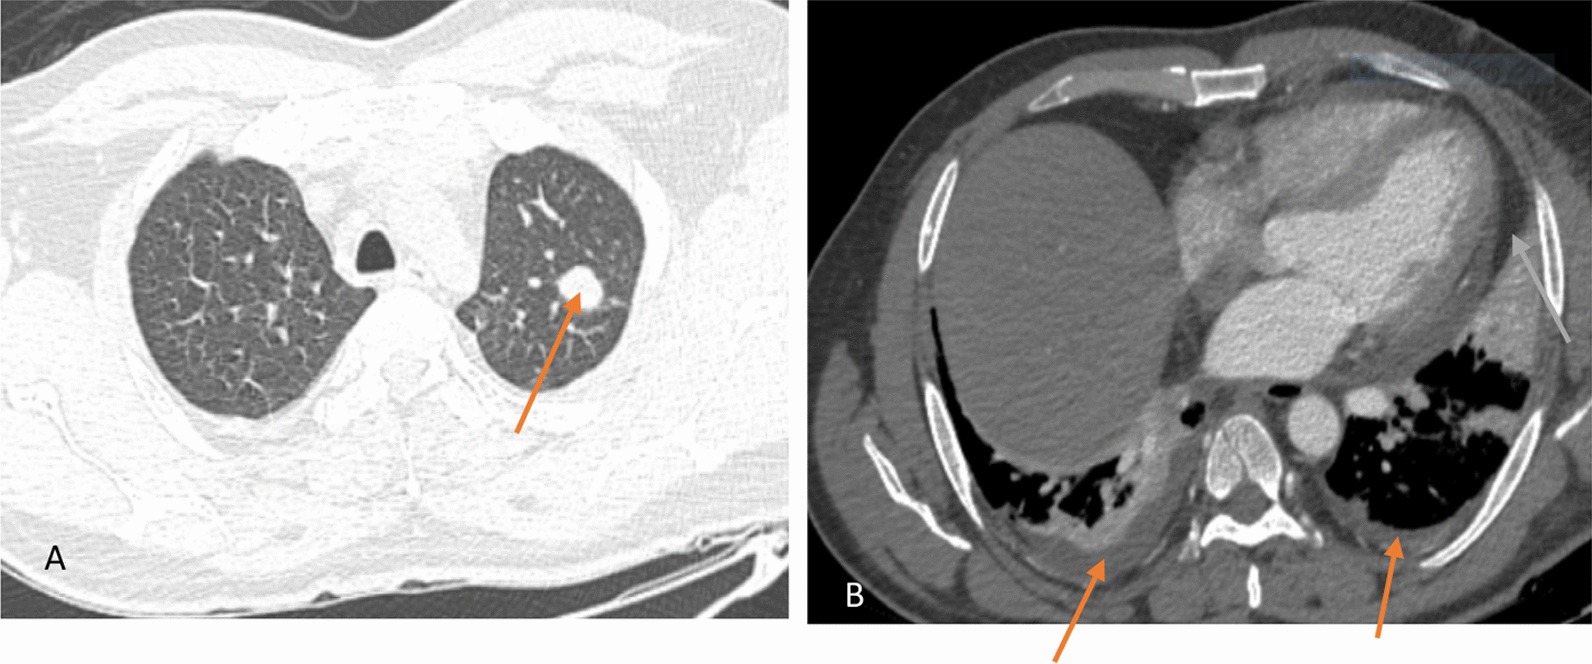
A Axial high-resolution CT (HRCT) image shows well-defined nodule in left upper lobe (red arrow). B Axial contrast-enhanced CT (CECT) chest shows bilateral pleural effusion (red arrows) and pericardial effusion (white arrow)
